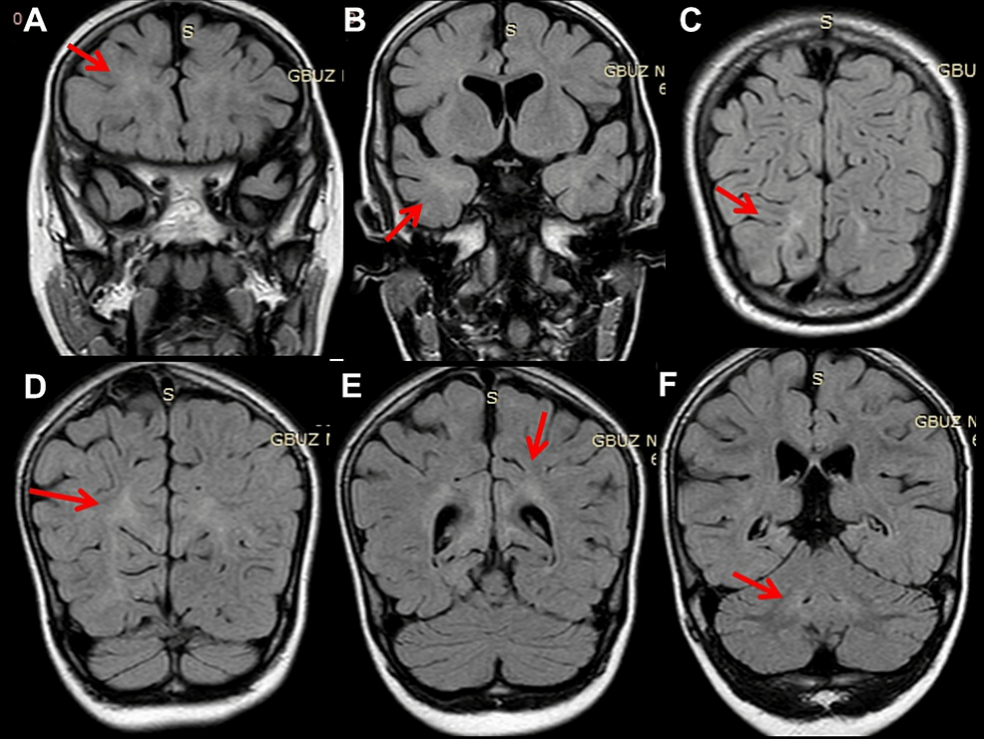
Cerebral MRI, fluid-attenuated inversion recovery (FLAIR) images, at age 27 months, showing hypomyelination supratentorially (A-C) and infratentorially (D-F), with right-sided predominance
